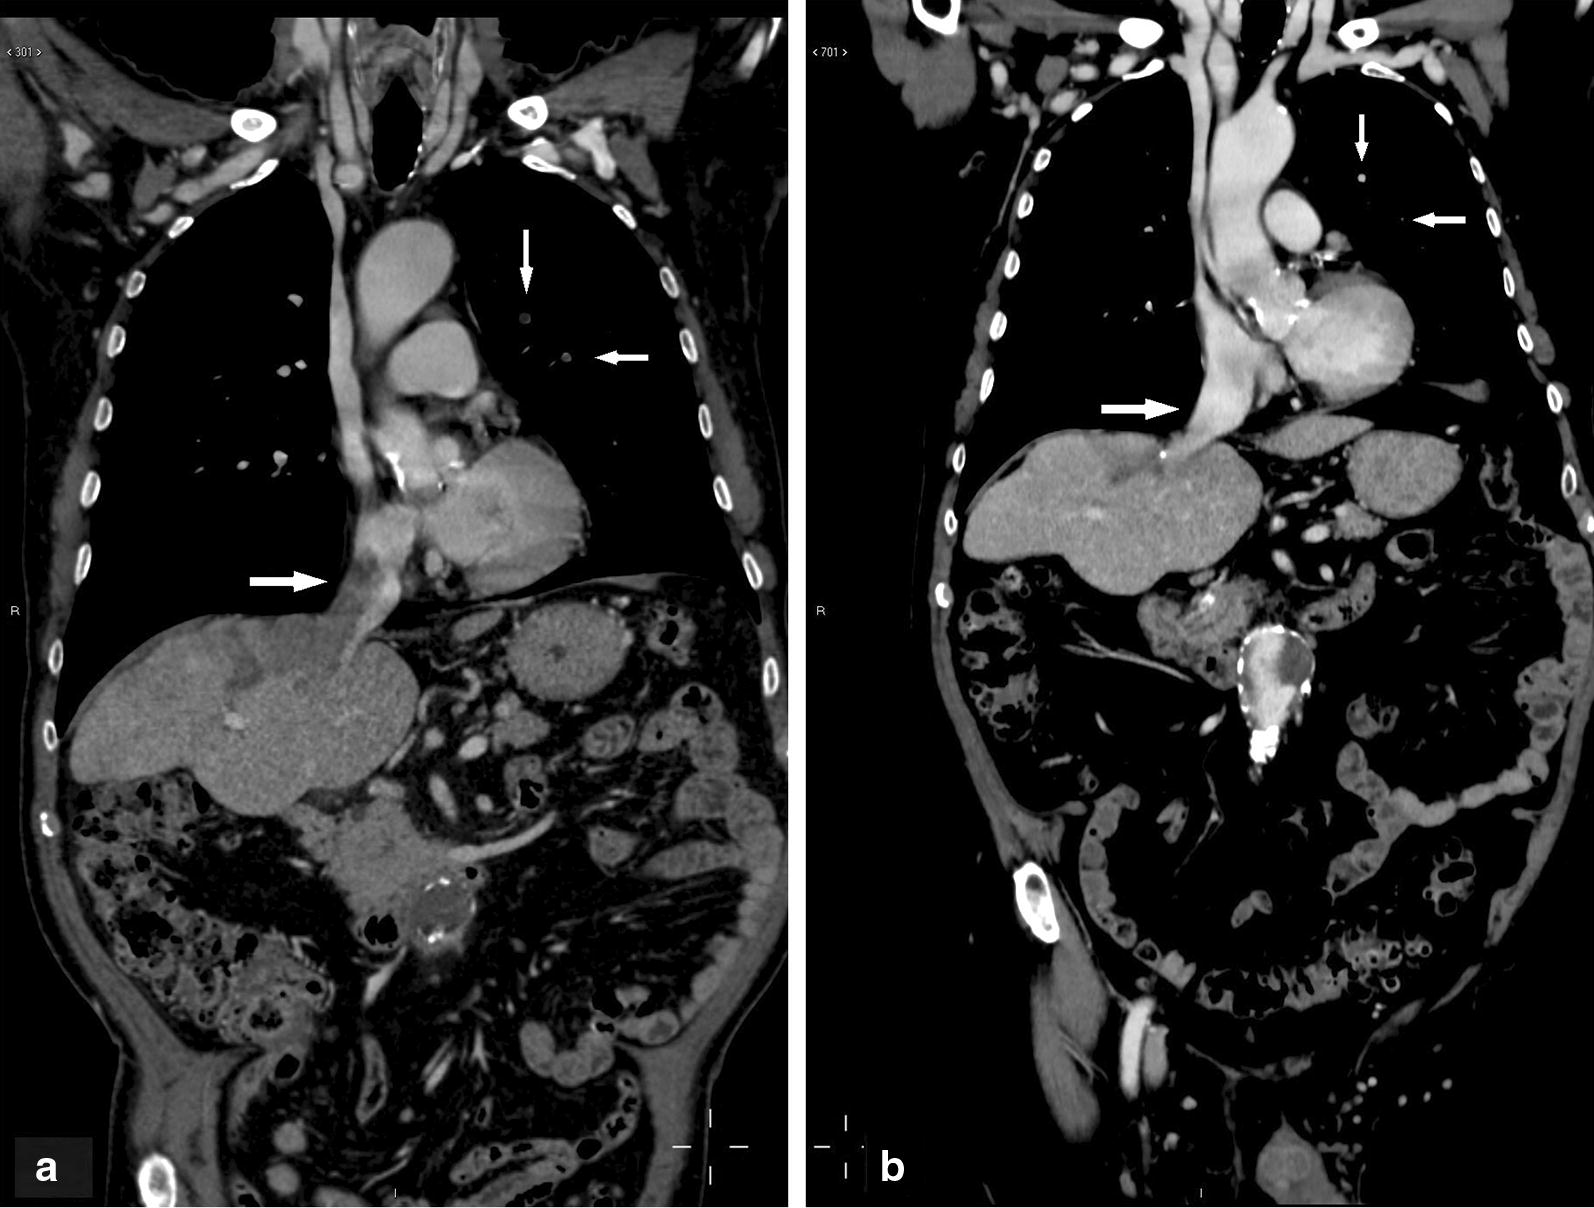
Lesions in case 3: a pre-treatment CT scan (coronal image of the portal fase). The image shows vascular invasion of the right and medium hepatic veins and of the inferior cava vein. Two neoplastic embolisms are present in the left lung. b Following 18 months of treatment with metronomic capecitabine, vascular vein invasion was resolved and pulmonary embolisms had disappeared
